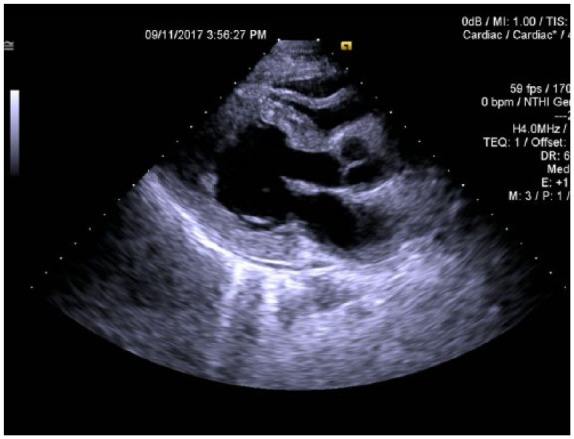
Echocardiography showing dilated ventricle in systole.
